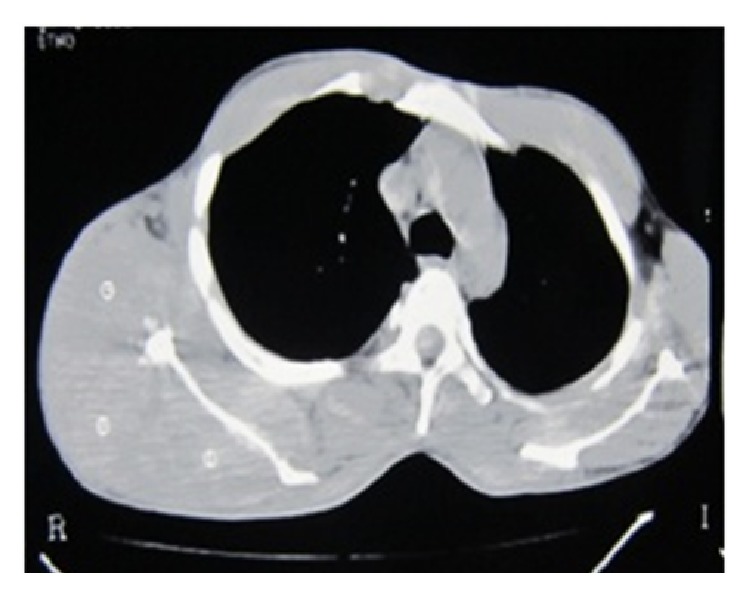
Contrast enhanced CT scan showing huge scapular swelling.
